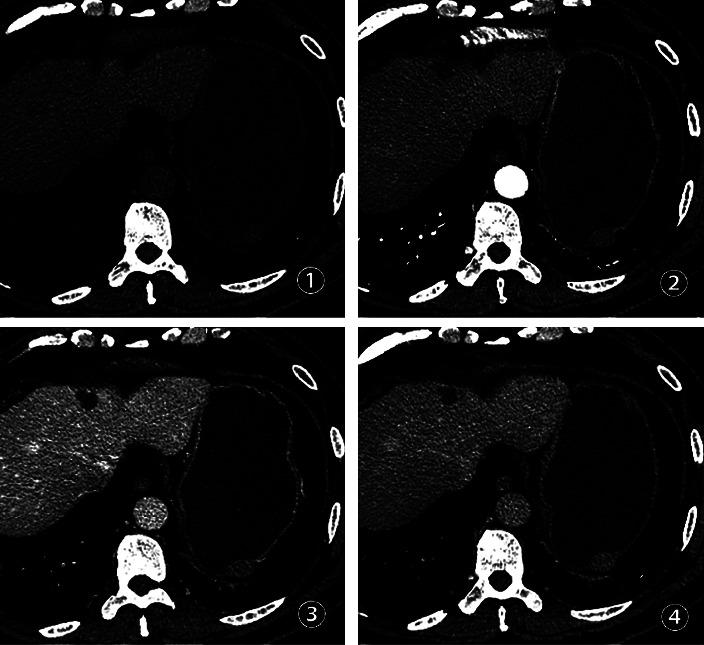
A 63-year-old man with small GISTs in the stomach wall. ① Plain CT scan revealing an oval mixed growth of soft tissue mass in the fundus of the stomach, smooth with clear margin and a maximum diameter of 2.1 cm. The CT value of plain CT scan was 32 Hu, showing mixed growth in the intraluminal/extraluminal. ②–④ Enhancement scanning of the arterial phase, venous phase, and delayed phase, resulting in uniform progressive enhancement of the mass.
